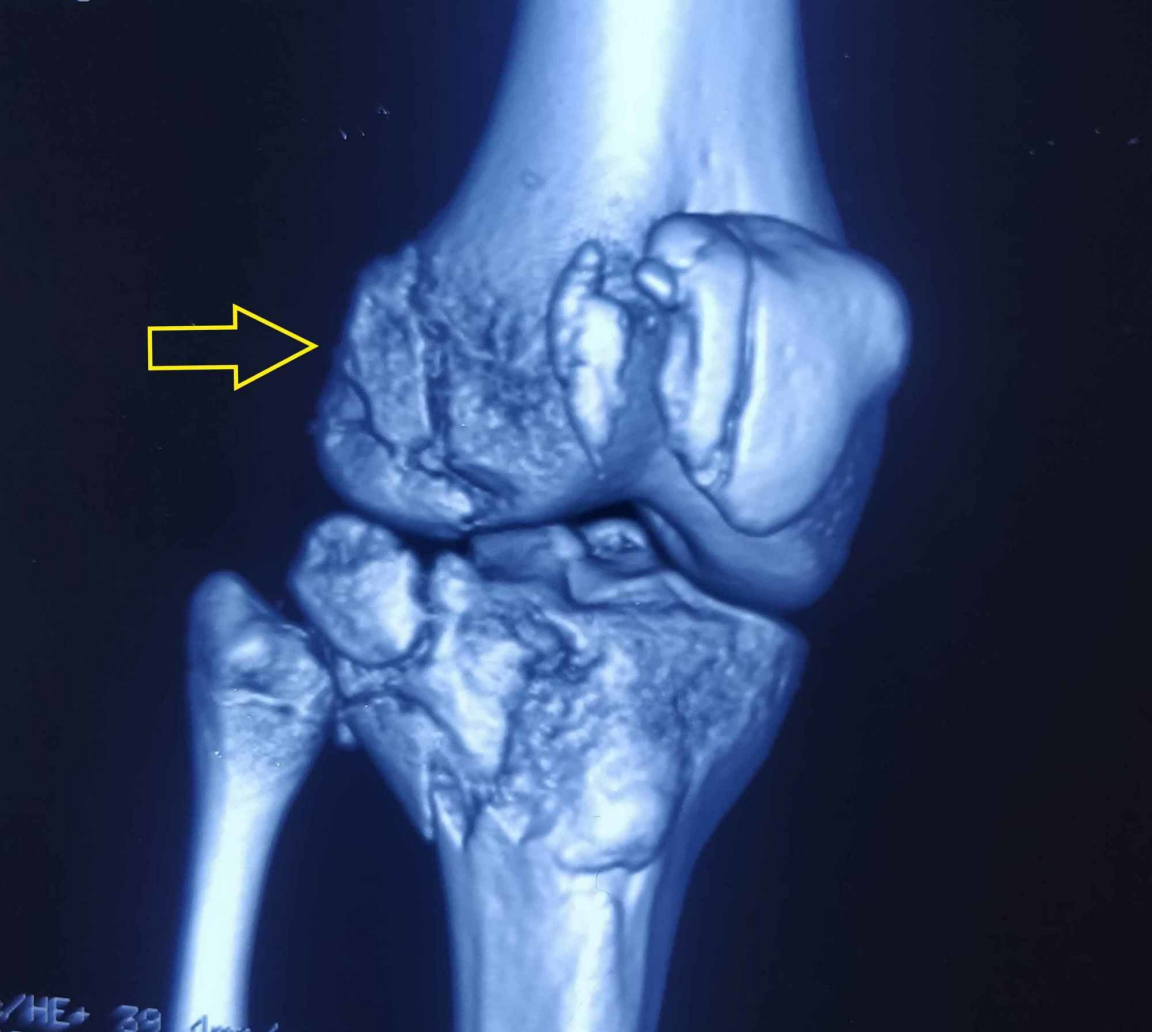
3D reconstruction image of CT scan showing femoral avulsion of posterolateral complex (yellow arrow)
